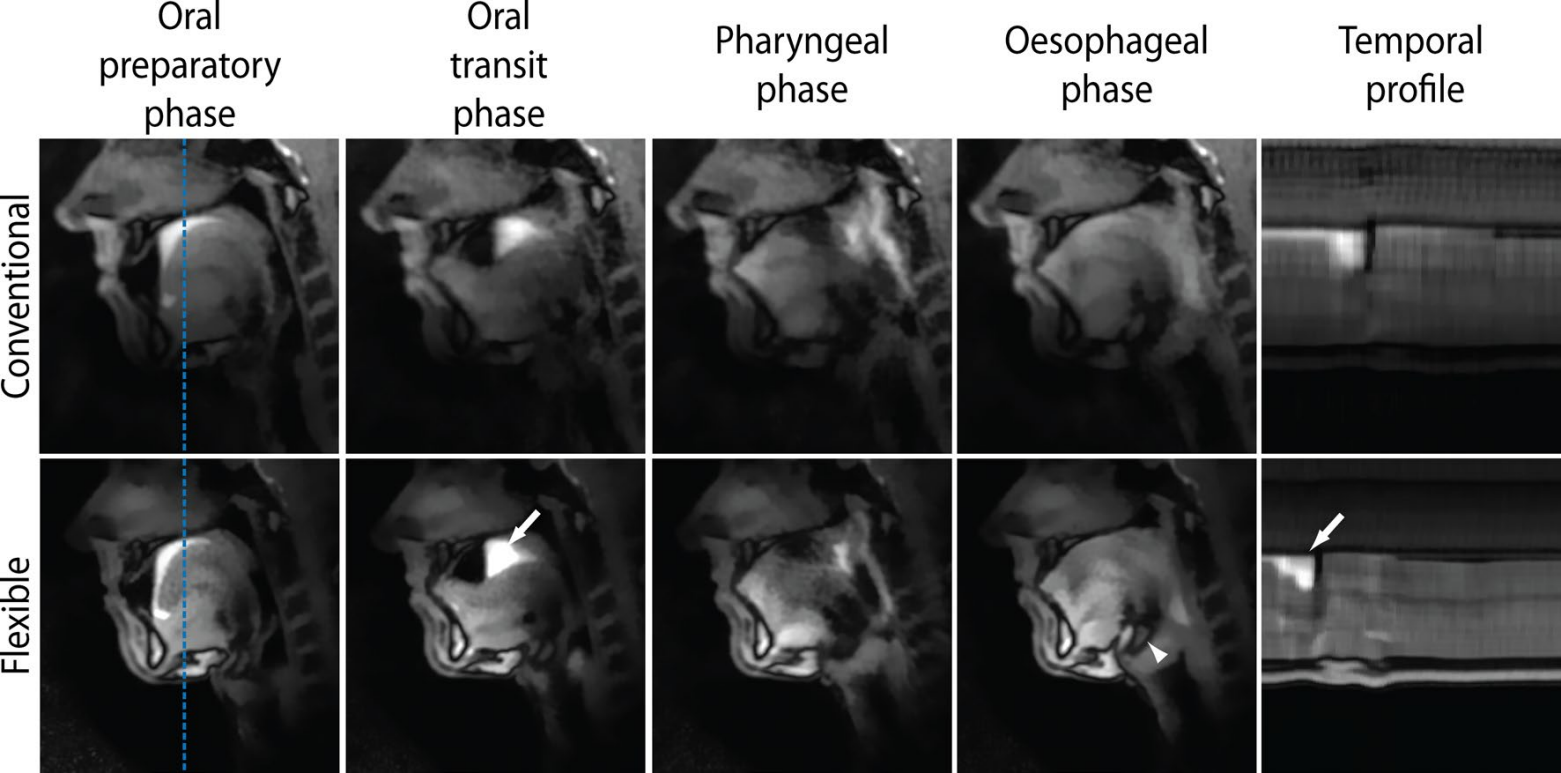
For the conventional and flexible coil, real-time MRI of swallowing at 43 frames per second is displayed for one volunteer. Four swallowing phases were distinguished: the oral preparatory phase, the oral transit phase, the pharyngeal phase, and the oesophageal phase. In the rightmost panels, the temporal profile of a single line, indicated by the blue dashed line, is shown. The white arrows, in the panels of the oral transit phase and the temporal profile for the flexible coil, indicate the swallowing contrast agent (pineapple juice). The white arrowhead, in the panel for the flexible coil in the oesophageal phase, indicates the hyoid, which could be significantly better visualised with the flexible coil (*P* = 0.029). The full movies of this volunteer are available for the conventional coil (Online Resource 1) and the flexible coil (Online Resource 2)
